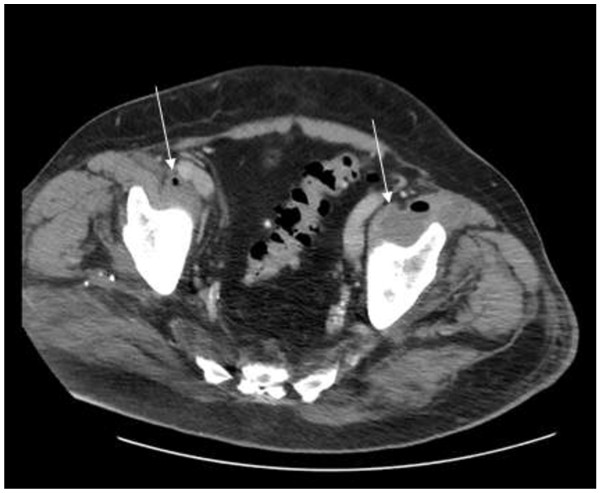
Transverse view on CT of the bilateral iliopsoas abscesses.
